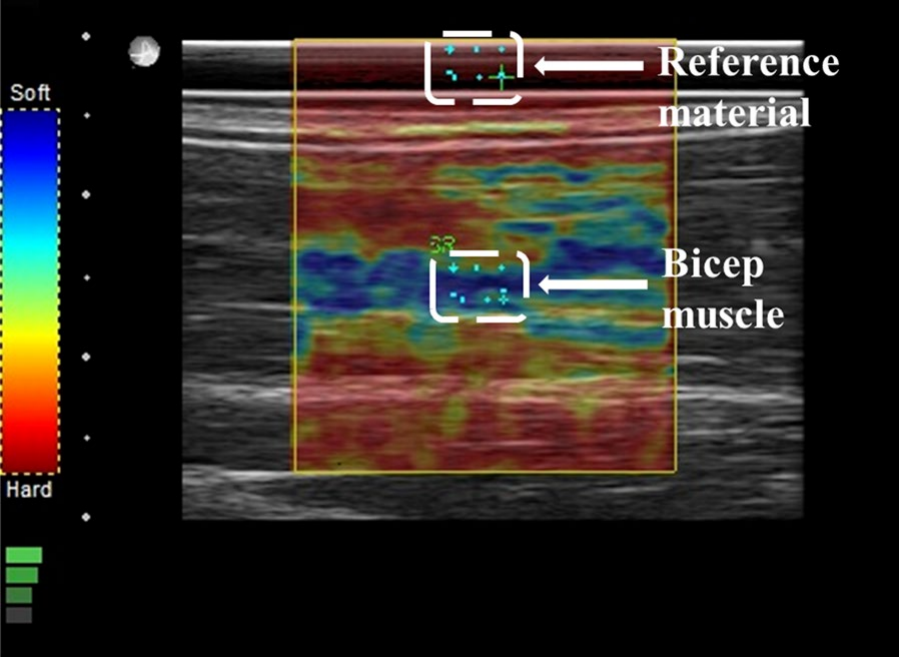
Ultrasonography measurement of bicep brachii muscle strain ratio using the reference material
